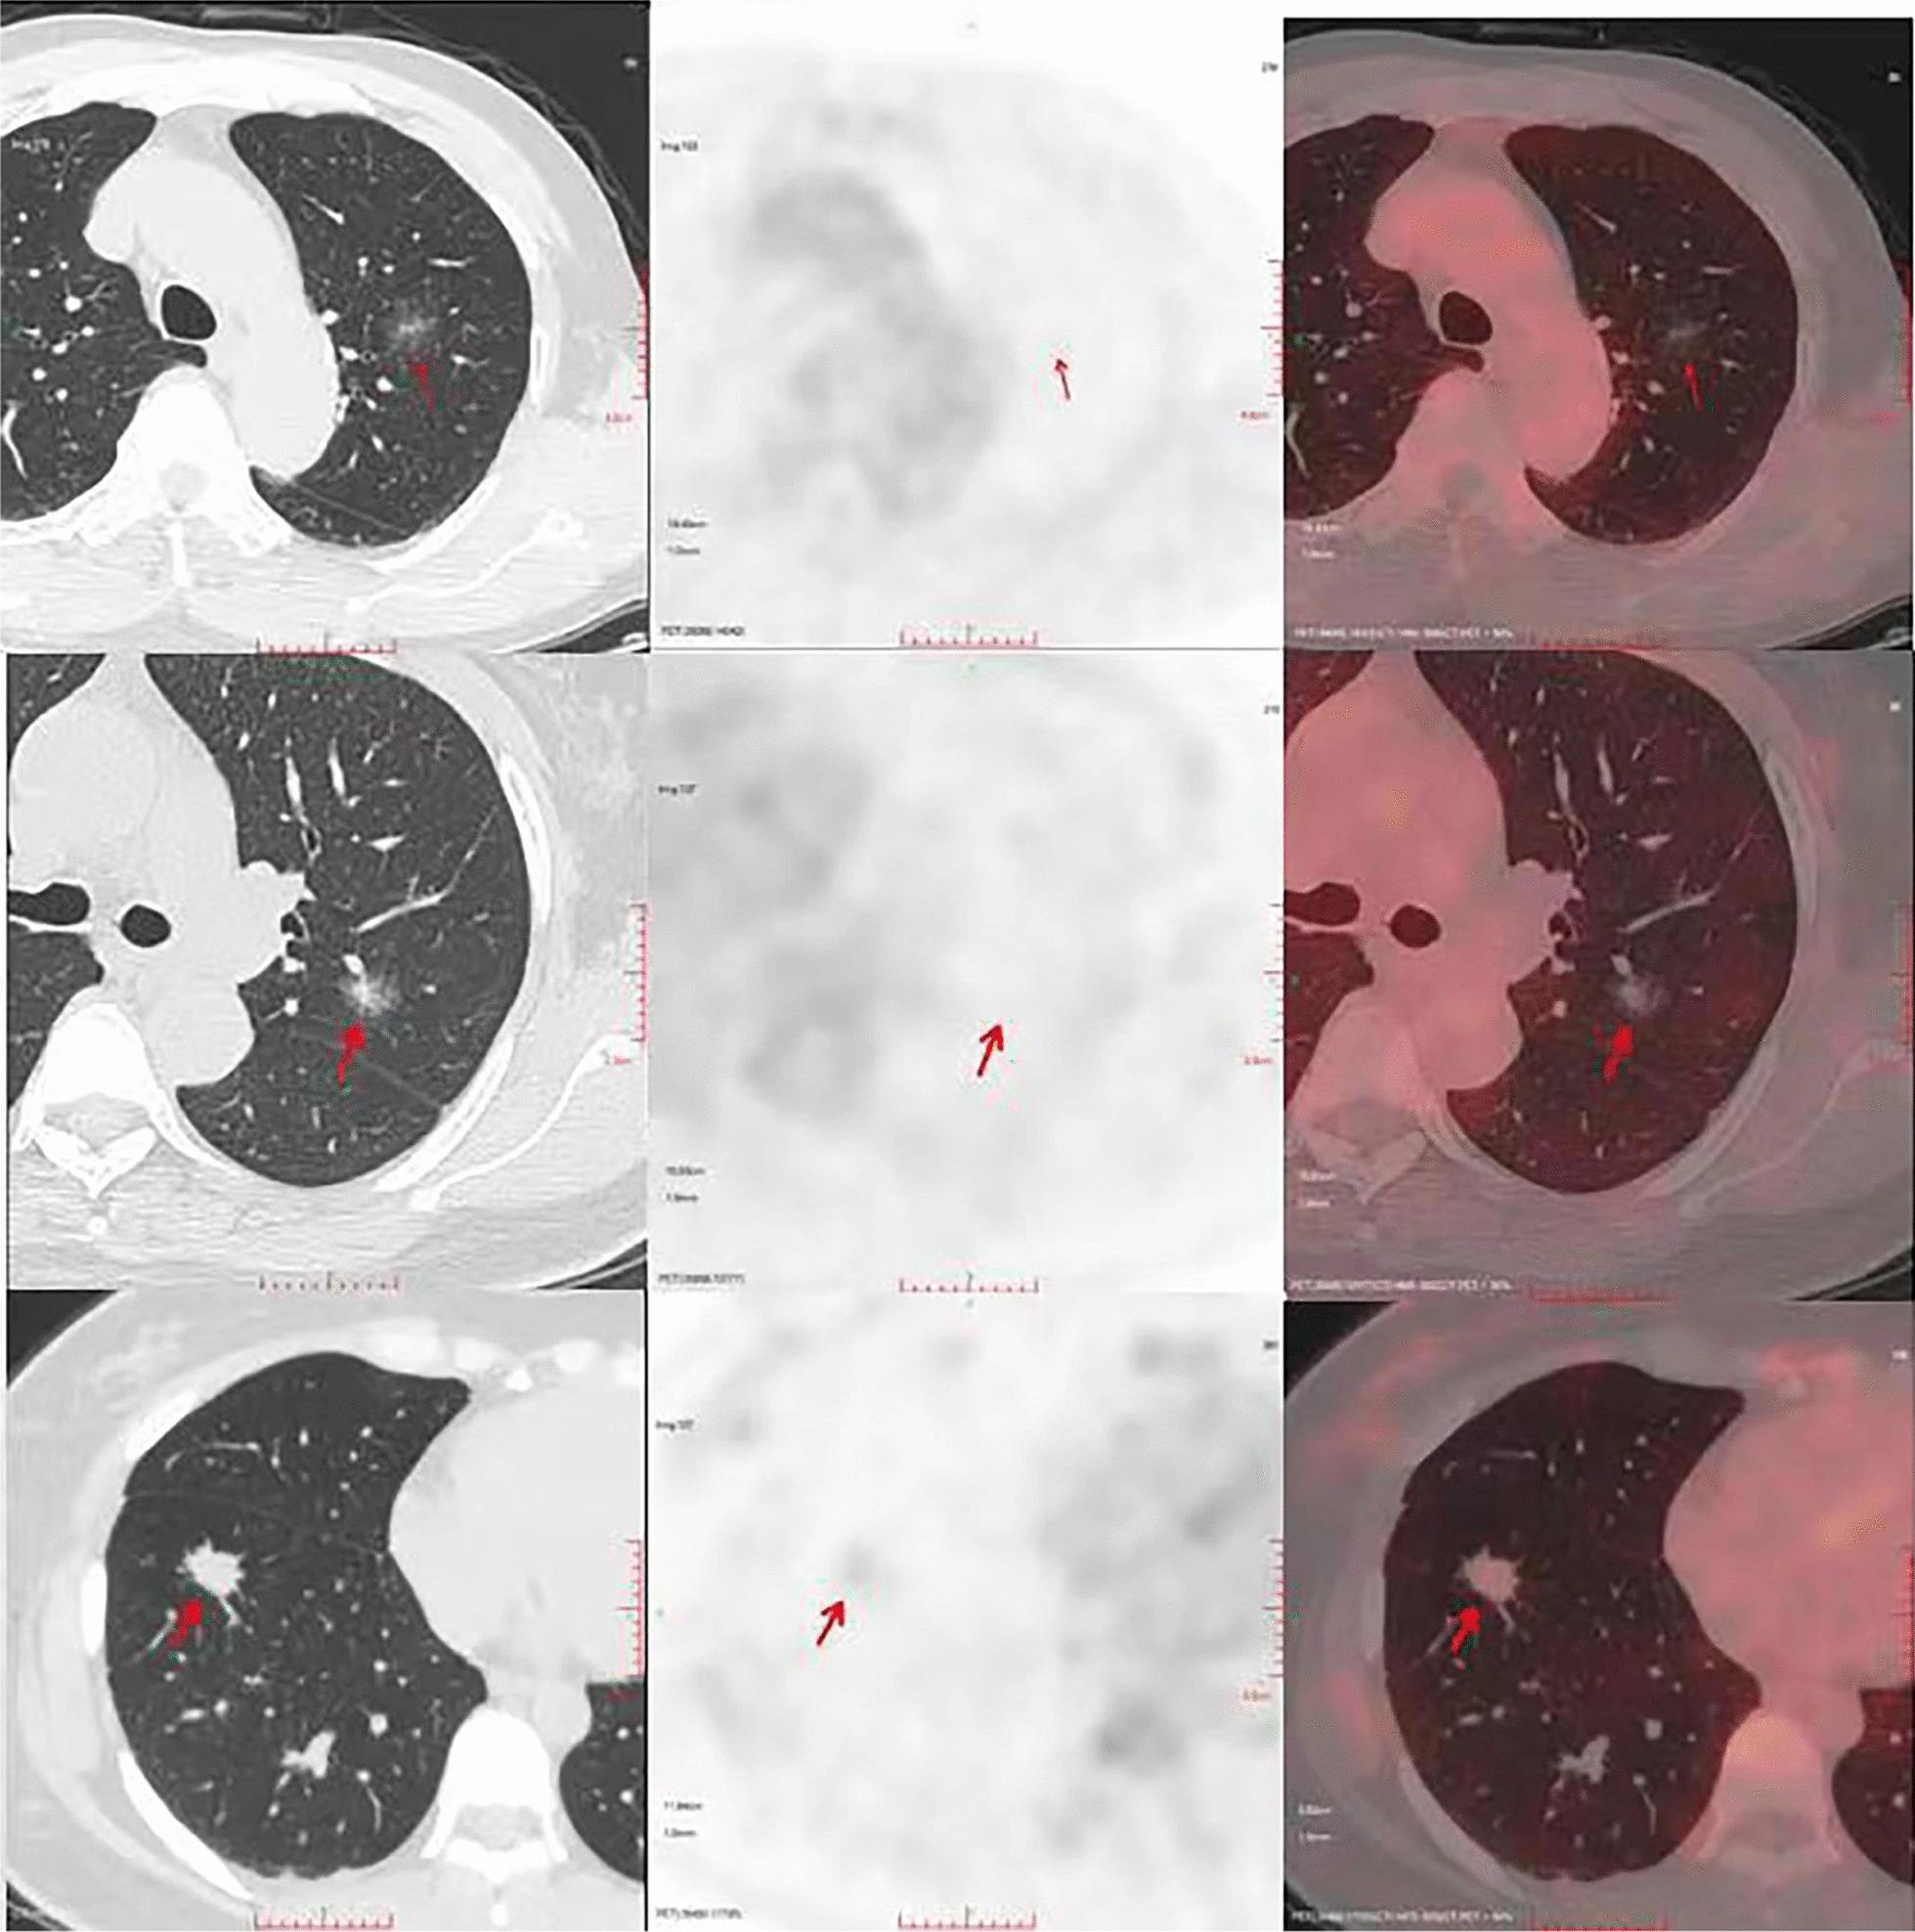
The representative images of MIA and IAC. MIA (the first row pictures show a mixed ground glass nodule in the upper lobe of left lung with no FDG uptake). IAC (the second row pictures show a mixed ground glass nodule in the upper lobe of left lung with no FDG uptake). IAC (the third row pictures show a solid nodule in the lower lobe of right lung with mild uptake of FDG, and the SUVmax is 1.5)
